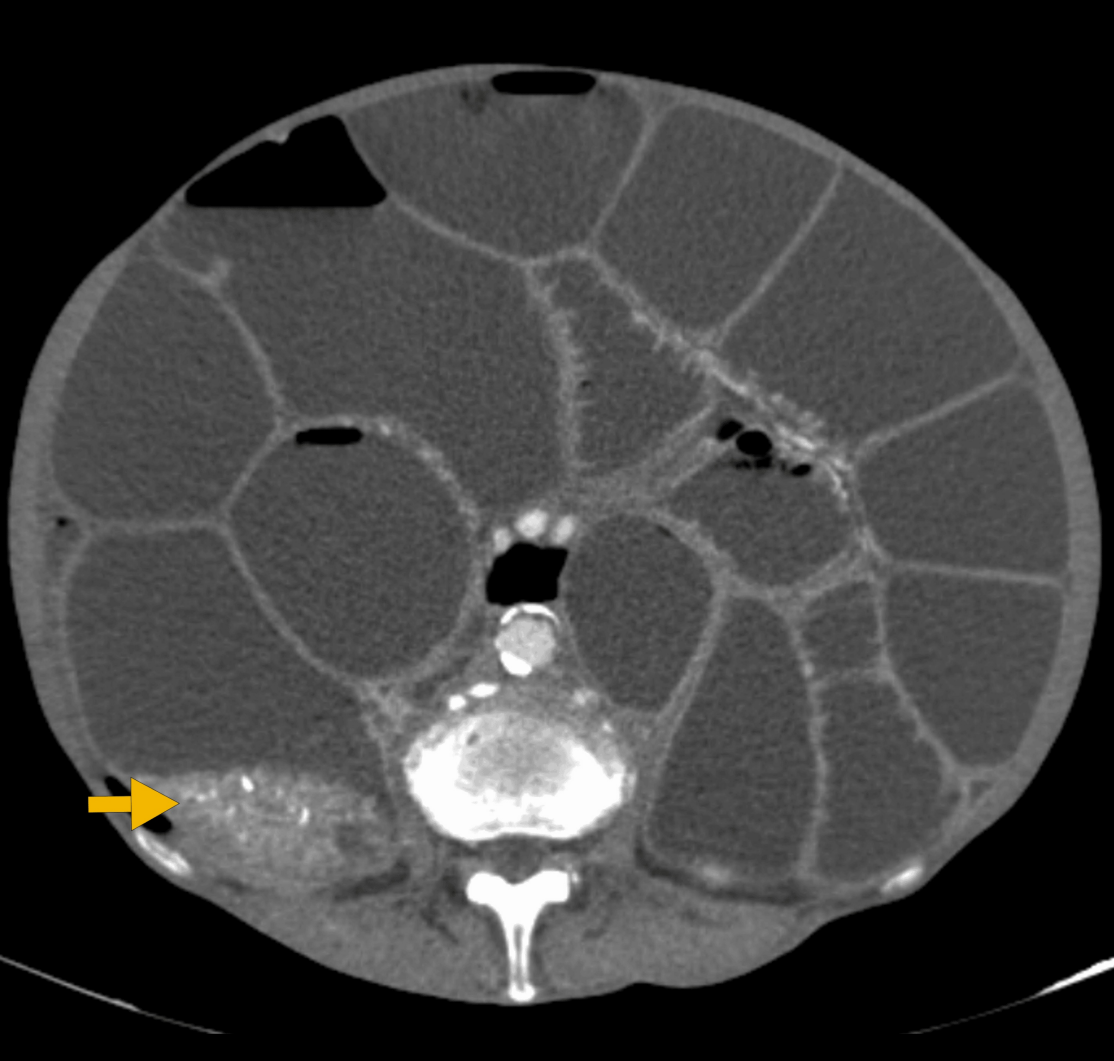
Abdominal and pelvic CT scan revealing hypervascular thickening at the level of the ileocecal valve (arrow), corresponding to the transition point. CT: Computed tomography
